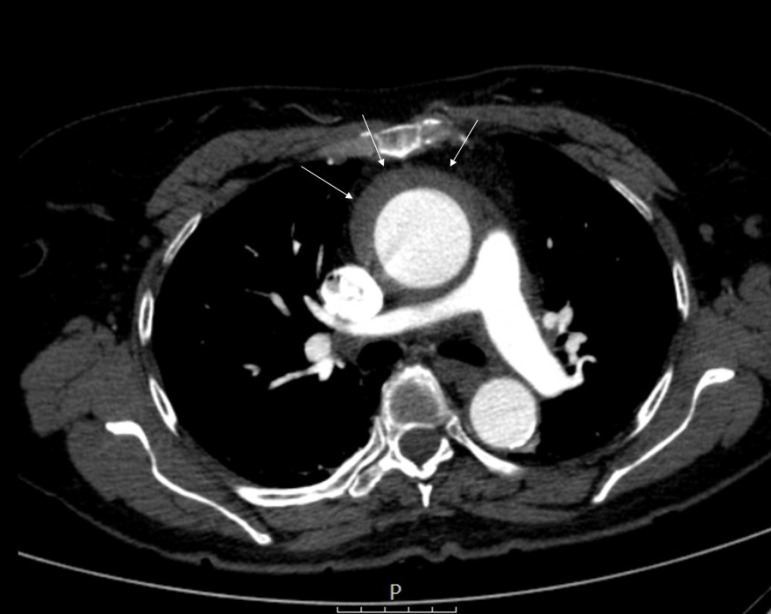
Computed tomography angiographic image showing type A intramural
